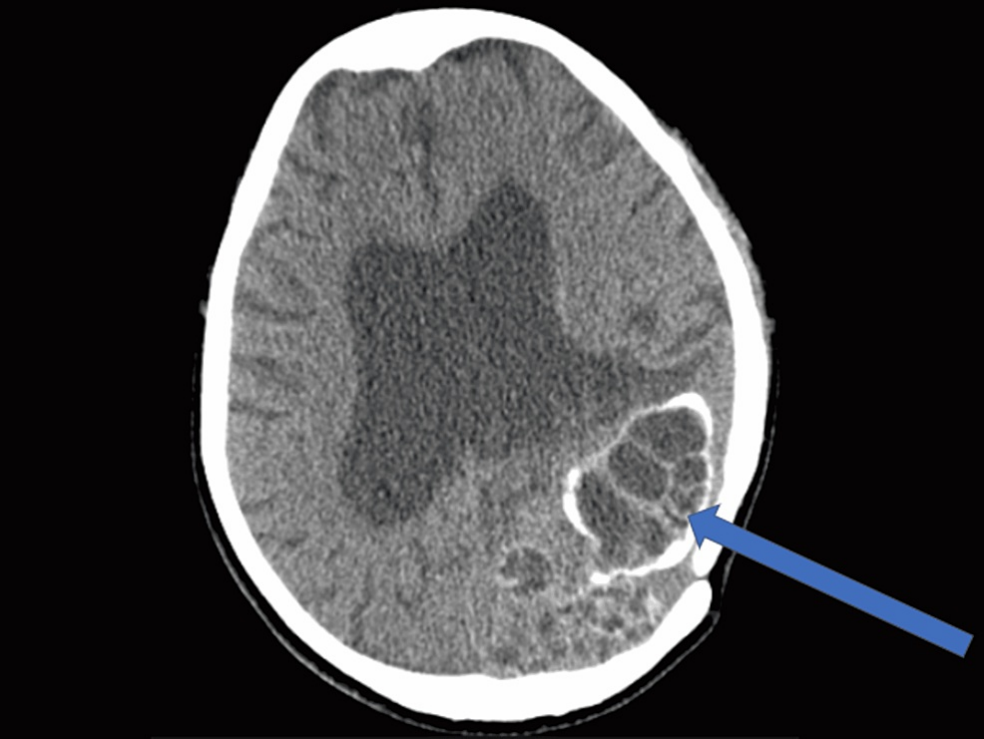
CT scan showing axial image of multiple intracranial multivesicular cysts in the left parieto-occipital region with localized calcifications (arrow).
